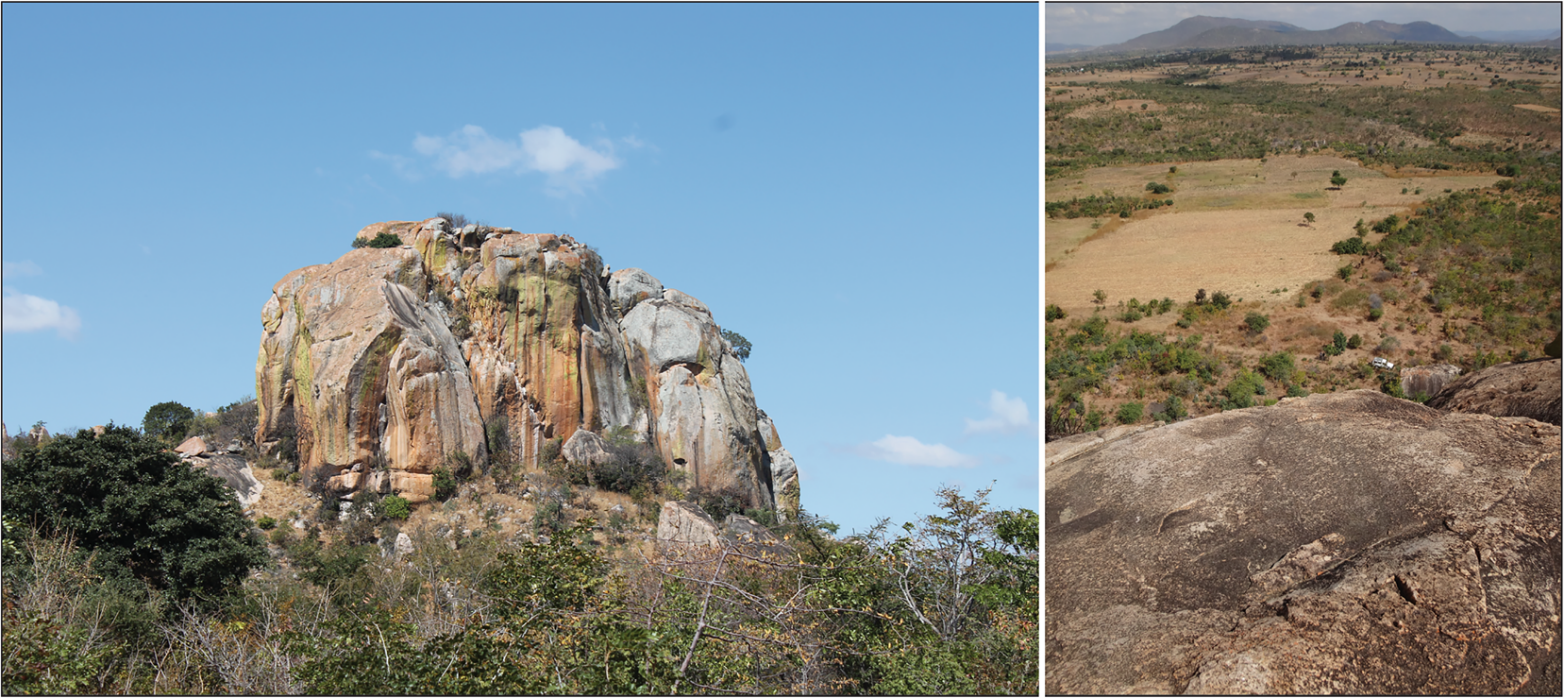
Upper portion of Mlangali Hill (left); looking down from a lower terrace, note the white SUV for scale (right)
